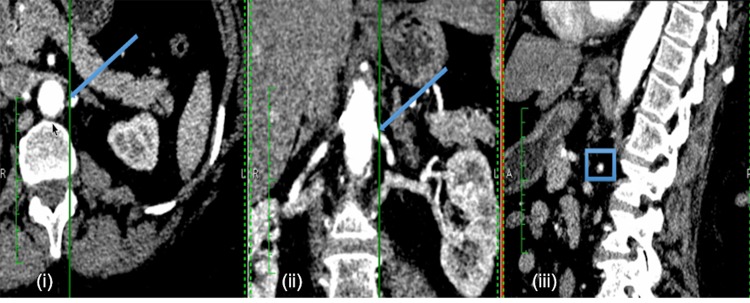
Example of patient-specific CT images. Example CT images of each anatomical orientation for Patient 2: axial (i), coronal (ii), and sagittal (iii). The arrows in the axial and coronal slices, and the box in the sagittal slice indicate the approximate location of the renal stenosis for the specific case.
